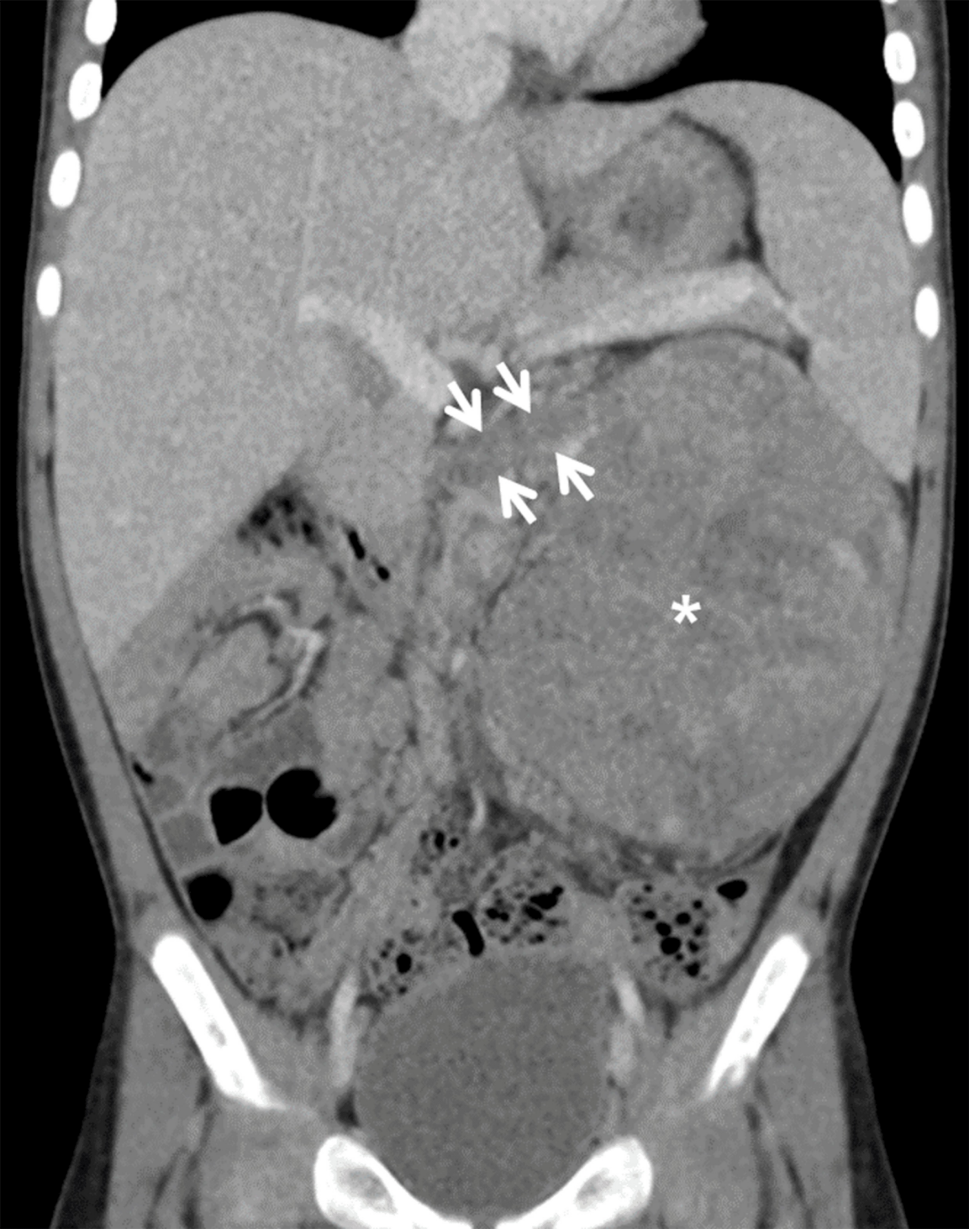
The coronal CT image shows WT in the left kidney (arrows) with a tumor thrombus extending to the left renal vein (asterisk). CT: computed tomography; WT: Wilms tumor
